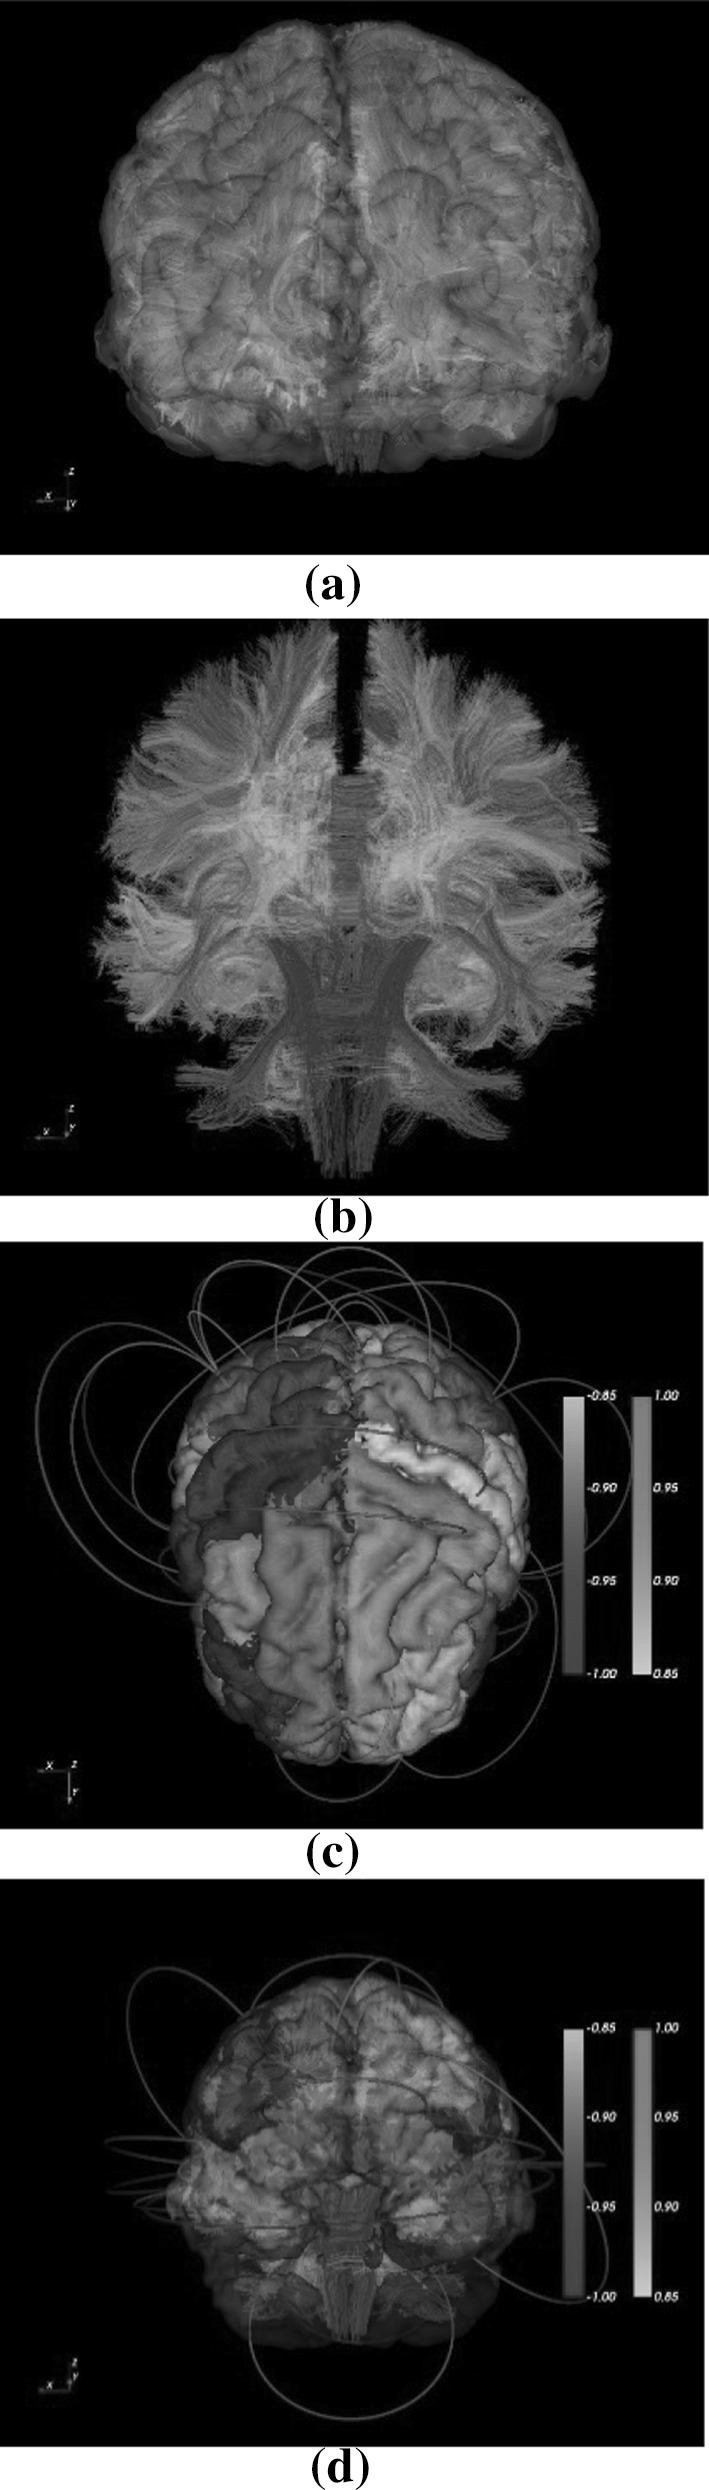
a DTI fiber tracts, b MRI-ROIs and DTI fibers, c, d network edges as Bezier curves (thresholded by edge intensity)
